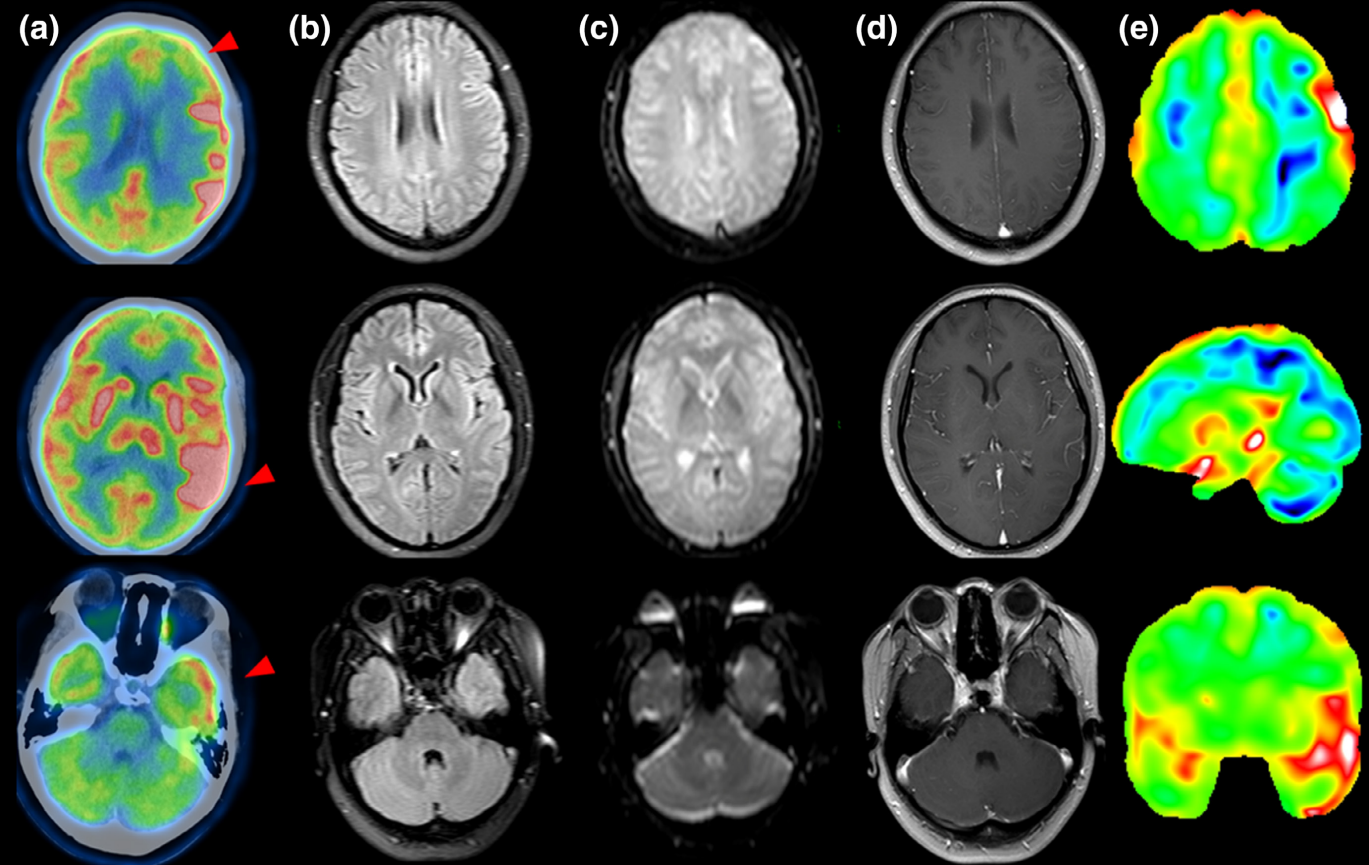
A typical case of the PET scan time is <1 month from symptom onset (pat. 6 had mental status changes and seizures for half a month, accompanied by cognitive impairment and speech disorders, and was diagnosed with ovarian teratoma). FDG‐PET in 21 days revealed that significant hypometabolism in left middle frontal, hypermetabolism in bilateral basal ganglia and left temporal lobe (A). However, cerebral MRI in 38 days shows unremarkable signal alterations on T2‐FLAIR image (B), DWI (C) and contrast enhanced MRI imaging (D). The FDG statistical deviation images analysis based on the *z*‐score (E).
